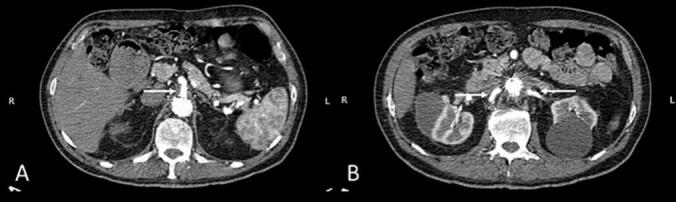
Axial post-procedural CTA image from Case 1 showing a thrombosed juxta-renal pseudoaneurysm adjacent to the fenestrated endograft. A) Patent celiac trunk and superior mesenteric artery are visualized arising anteriorly from the aorta. B) Bilateral renal arteries are patent and arise just proximal to the endograft.
